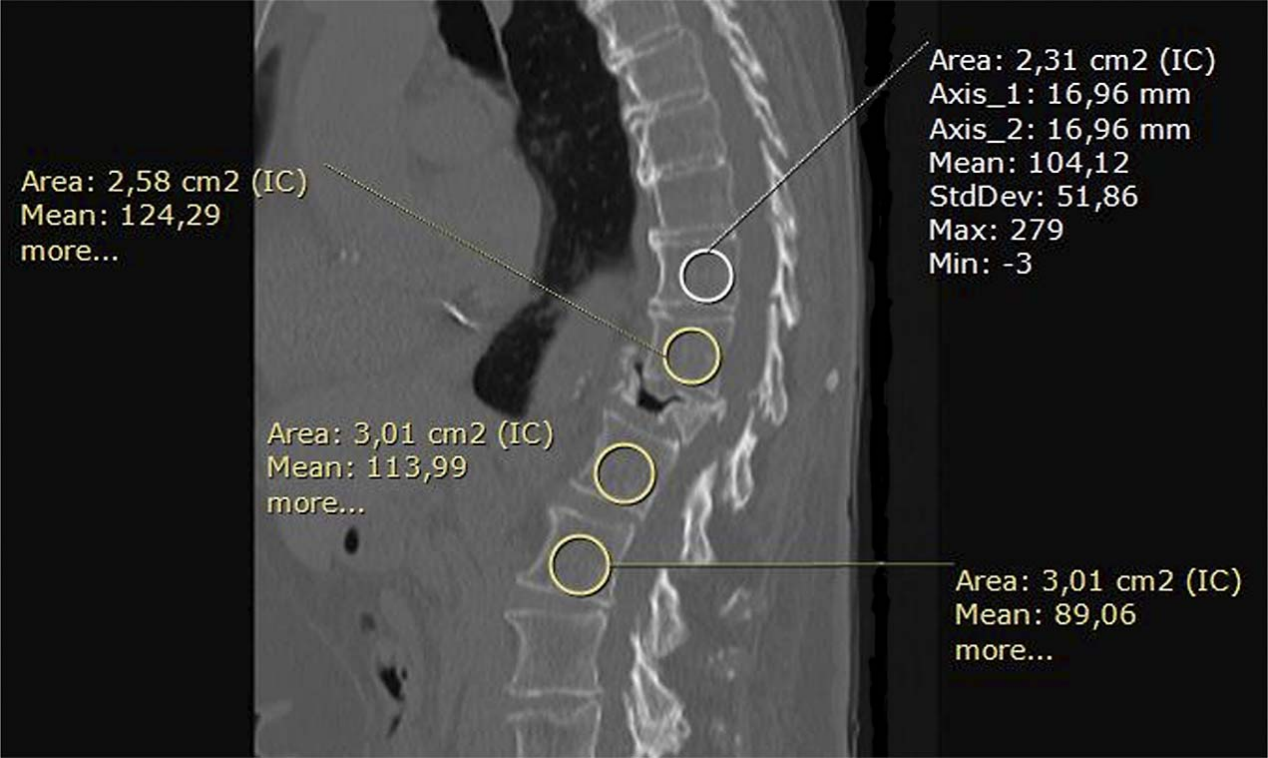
The sagittal view of the computed tomography shows the mean Hounsfield unit measurement of the spine levels (T10-L2) planned to be included in an instrumentation because of an osteoporotic fracture of T12.
